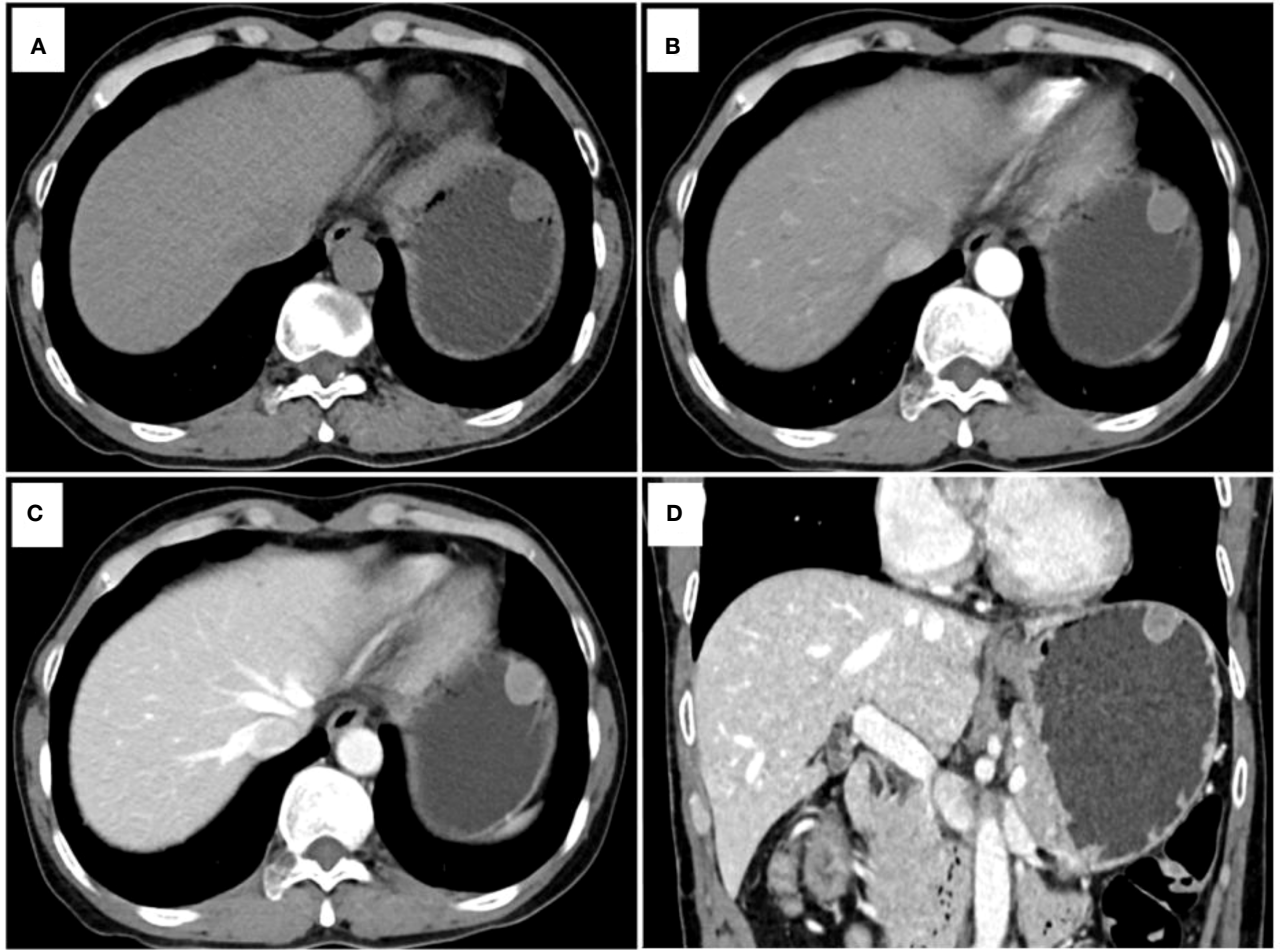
Another example of a point-based scoring system in use. CT examination of patient 2, including axial unenhanced (A), arterial phase image (B), venous phase image (C), and coronal venous phase image (D), showed an endoluminal growth pattern lesion in the gastric fundus without tumor surface ulceration. The CT attenuation value of the tumor in the venous phase images (Value_TV_) and the tumor-to-spleen ratio (TSR) were 79 HU and 0.54, respectively. The total risk score of gastric schwannoma (GS) was calculated to be 0 points with a low probability of 2.00% in the point-based scoring system (PSS). Pathology reports showed that the tumor was a gastrointestinal stromal tumor (GIST).
